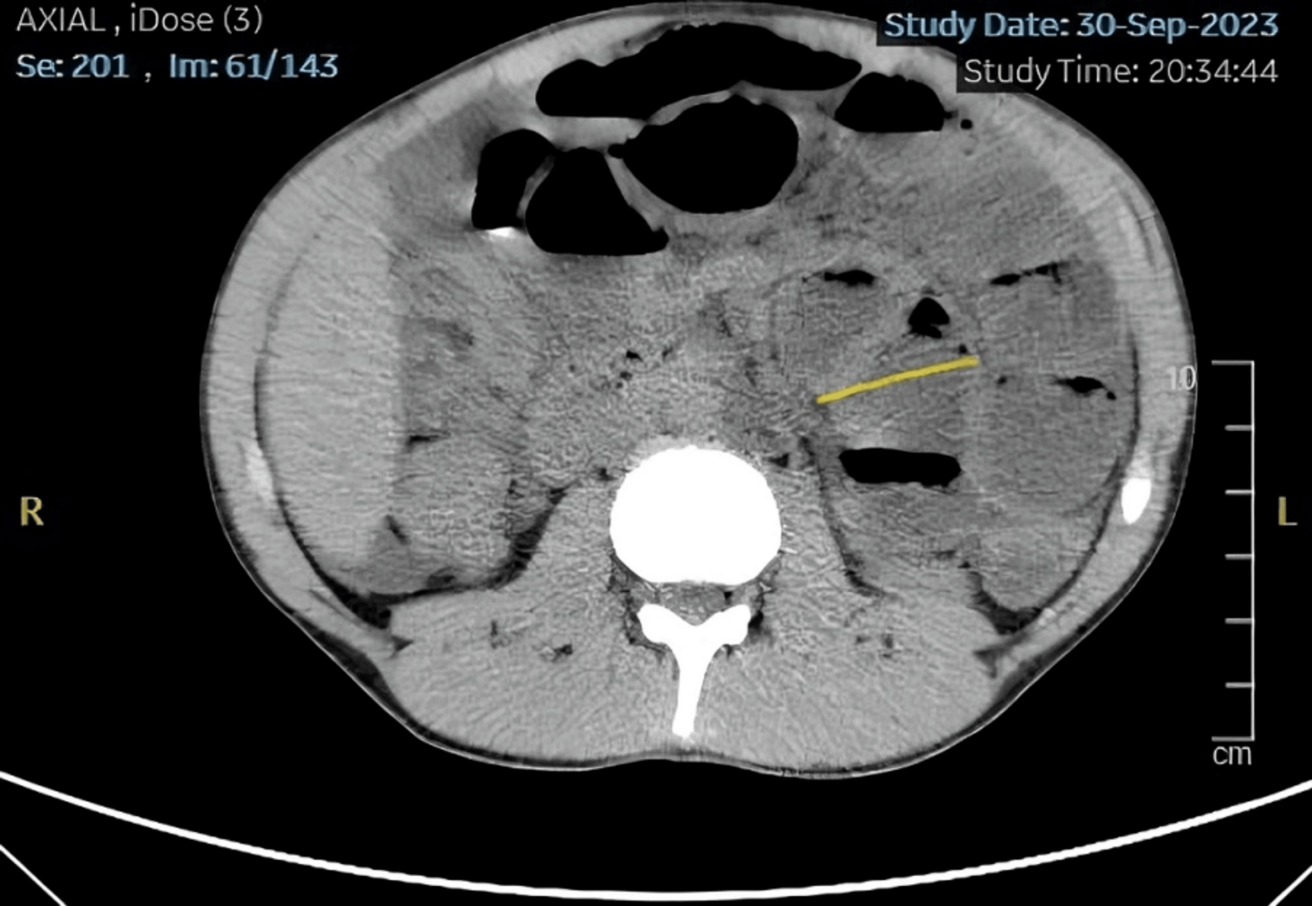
CT abdomen and pelvis. Dilated proximal small bowel loops in the left hemiabdomen (yellow line indicating dilatation up to 3.8 cm) with fluid-filled loops in the right hemiabdomen in a pattern consistent with small bowel obstruction.
